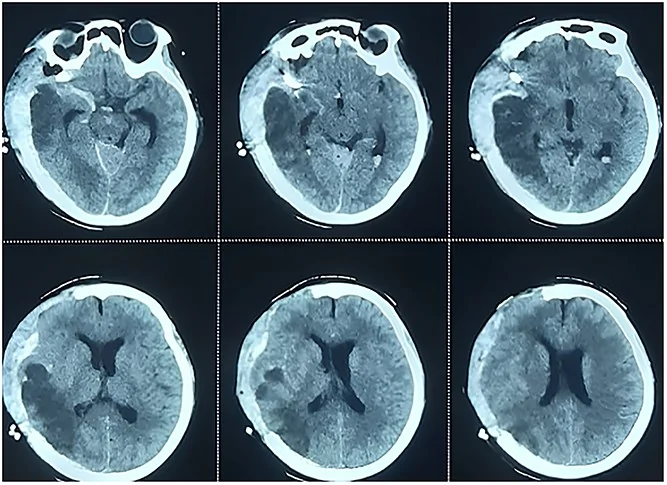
Post-operative CT brain images showing features of right-sided peri-opercular infarction.
